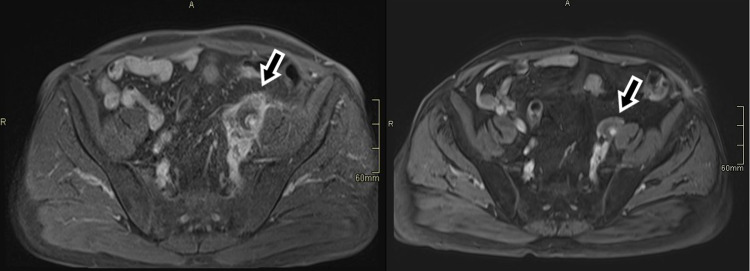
T1 sequence MRI of the abdomen in the axial plane when the patient was first admitted to the hospital (A), and a follow-up after two months of treatment (B). The retroperitoneal fibrosis is marked with arrows. MRI: magnetic resonance imaging
